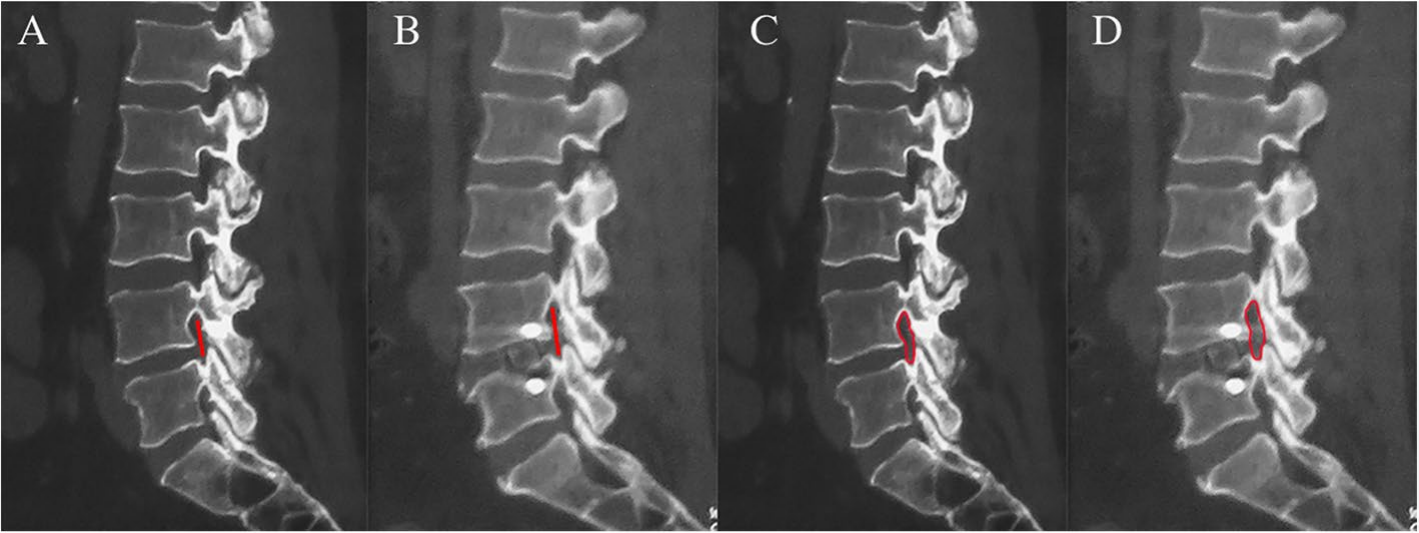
CT-related index measurement. A, B The L_4-5_ FH measured on preoperative (A) and postoperative (B) sagittal CT scan images: the shortest distance between the lower edge of the upper pedicle and the upper edge of the upper pedicle. C, D The L_4-5_ F-CSA was measured on preoperative (C) and postoperative (D) sagittal CT scan images
